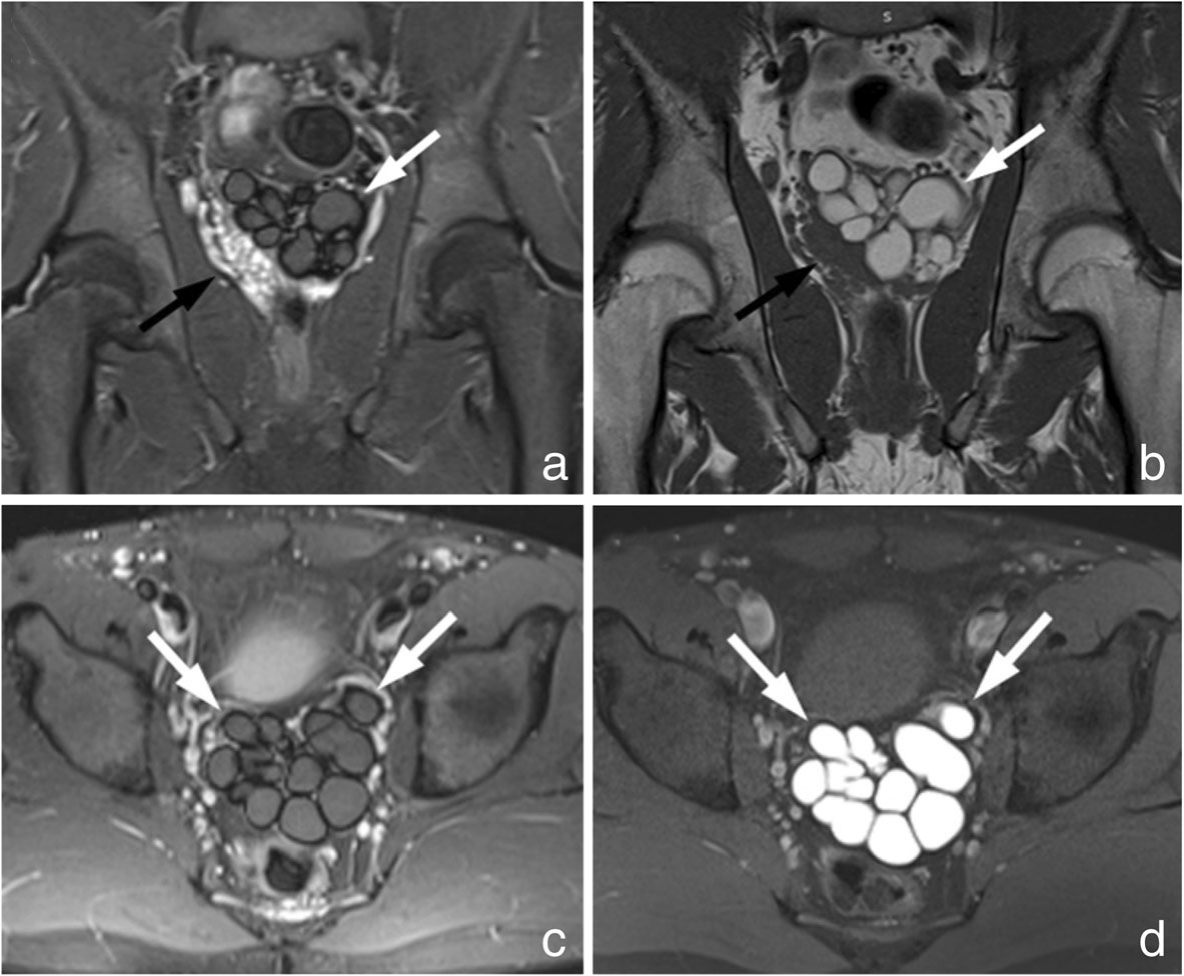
Coronal MR images demonstrate a large lobulated multicystic lesion in the anatomic region of left seminal vesicle with low signal intensity on T_2_-weighted FS images (a) and high signal on T_1_-weighted images (b), corresponding to a dilated SVC (white arrows). The multilobulated SVC is also depicted on axial T_2_-weighted FS (c) and T_1_-weighted FS (d) images with low signal and high signal intensity, respectively, suggesting proteinaceous or hematic content (white arrows). The normal right seminal vesicle is shown on coronal T_2_-weighted FS (a) and T_1_-weighted (b) images with high and intermediate signal intensity, respectively, corresponding to fluid (black arrows)
